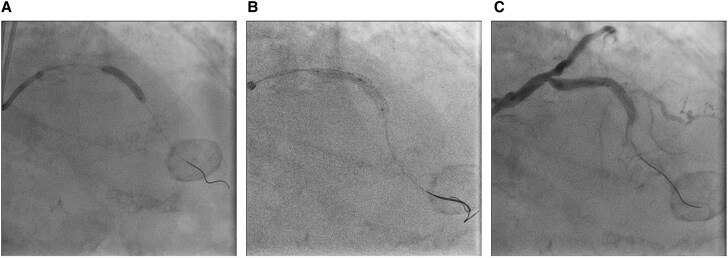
(*A*) A 3.5 × 16 mm drug-eluting stent implanted in the mid-left anterior descending artery. (*B*) Real-time intravascular ultrasound evaluation of the behaviour and dynamics of a semi-compliant 2.0 mm balloon. (*C*) A 4.0 × 18 mm drug-eluting stent intentionally deployed in the true lumen over a wire that had migrated into the subintimal space.
